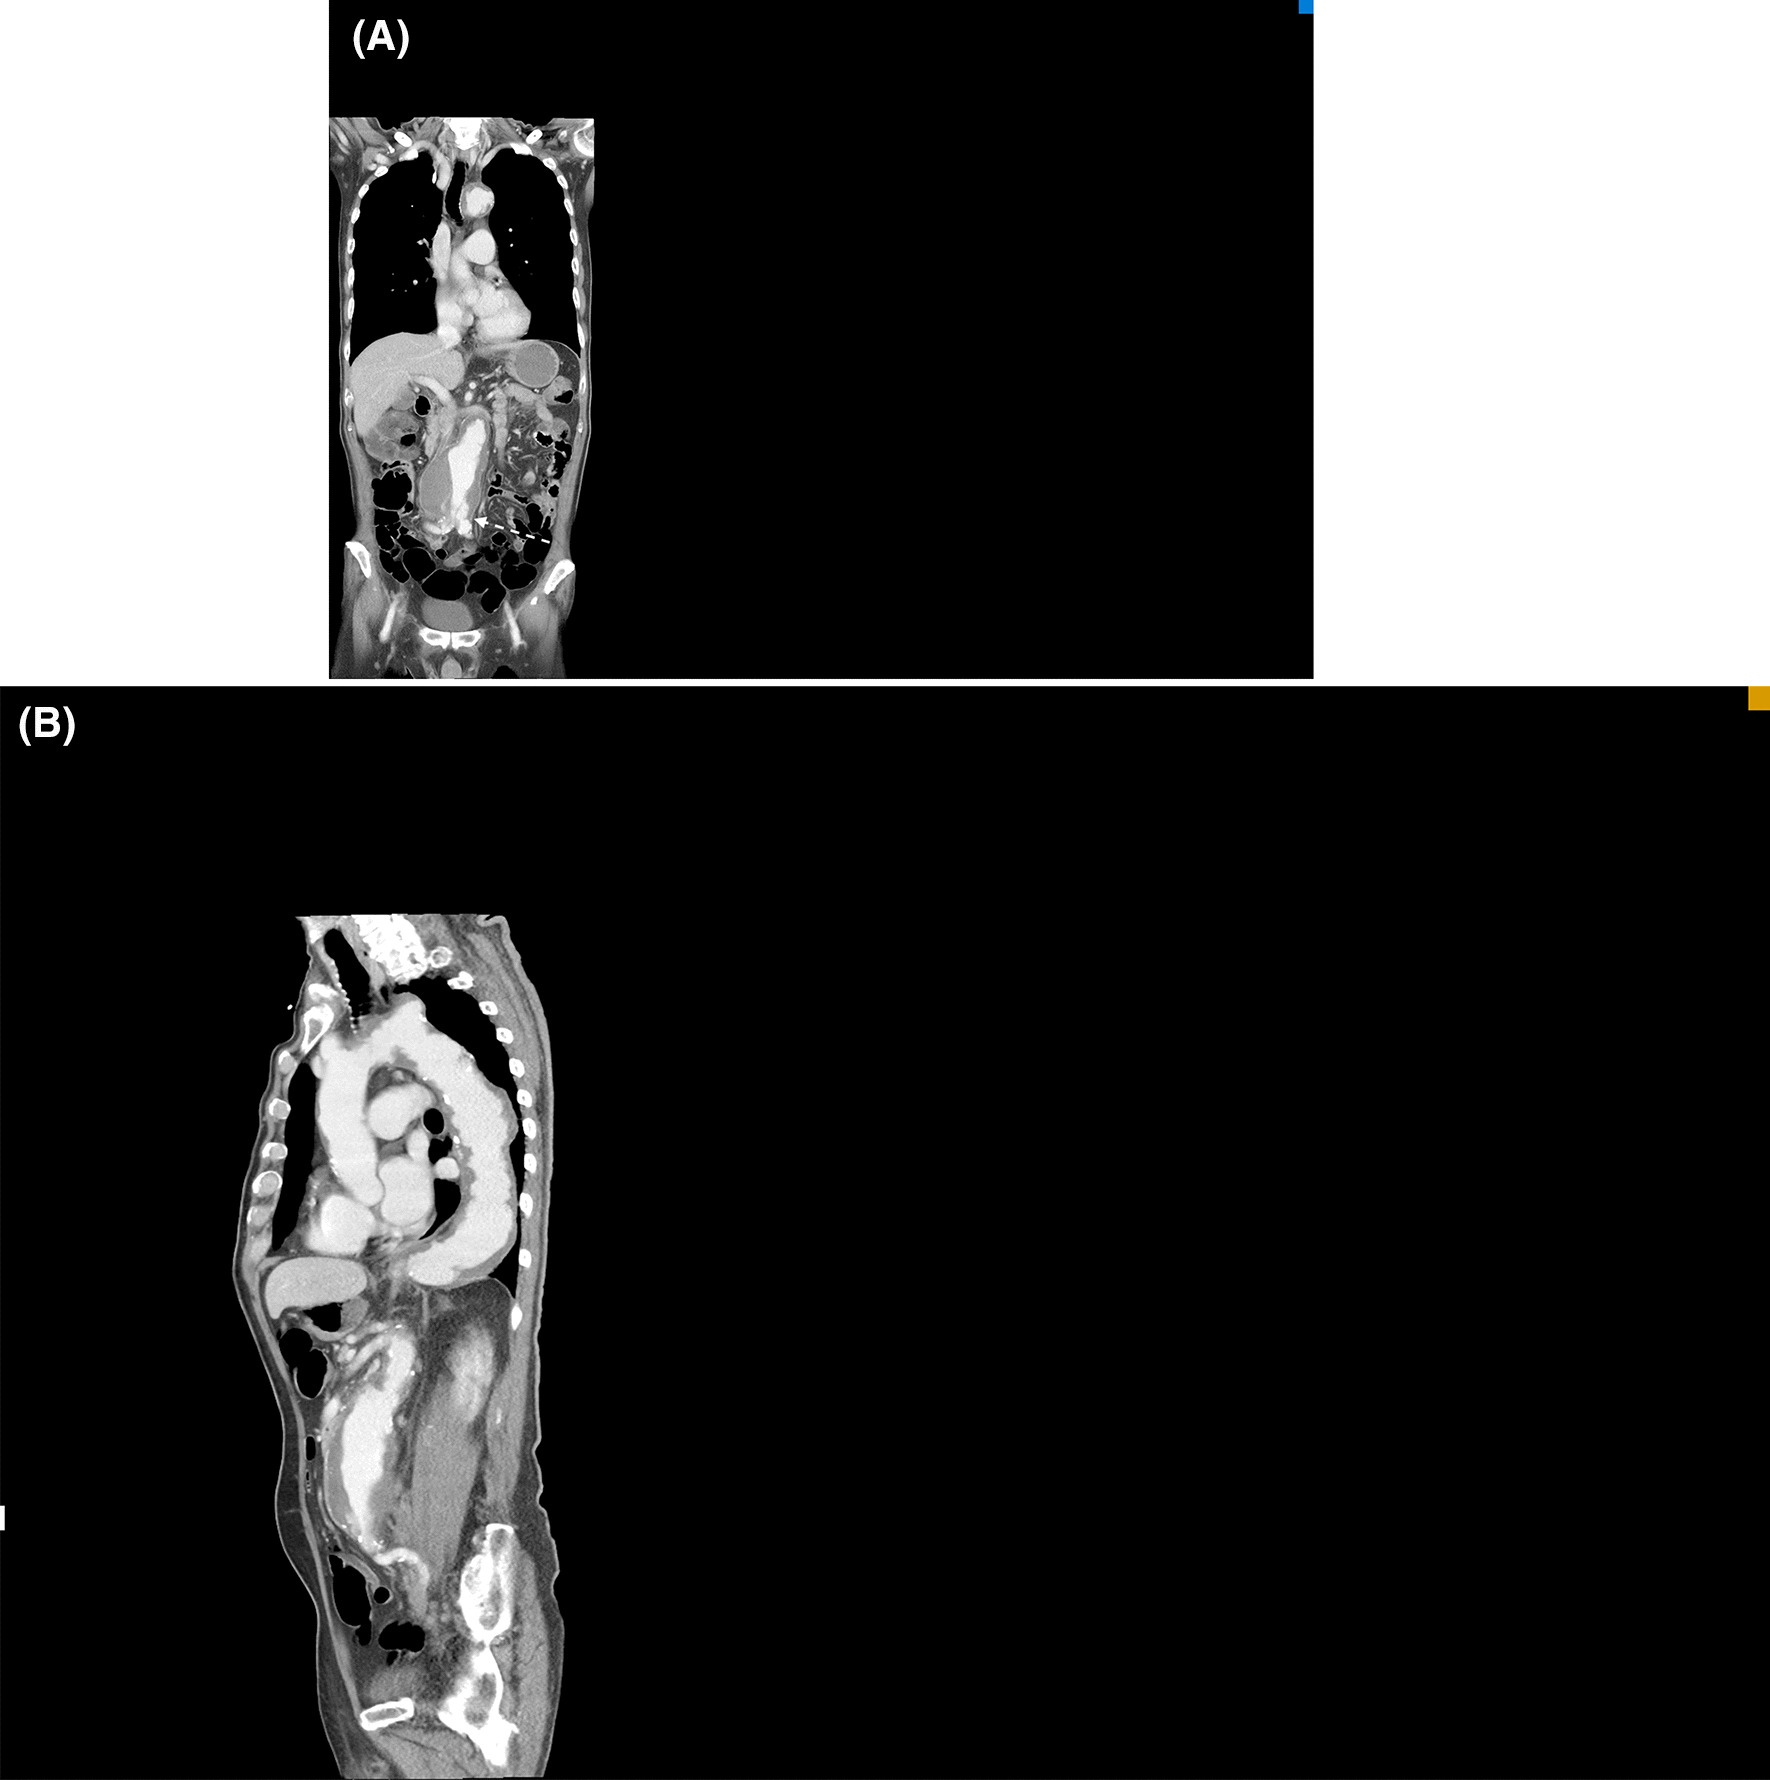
In the delayed phase of CTA, A contrast medium was detected in the venous system, shown in coronal view, and there was a signal was apparent in the common femoral arteries. B There was patent blood flow in the iliac arteries in which non-occlusive acute limb ischaemia was documented caused by poor inflow (The dotted arrow shows partial occlusion related to a floating flap of thrombus, without embolic occlusion)
